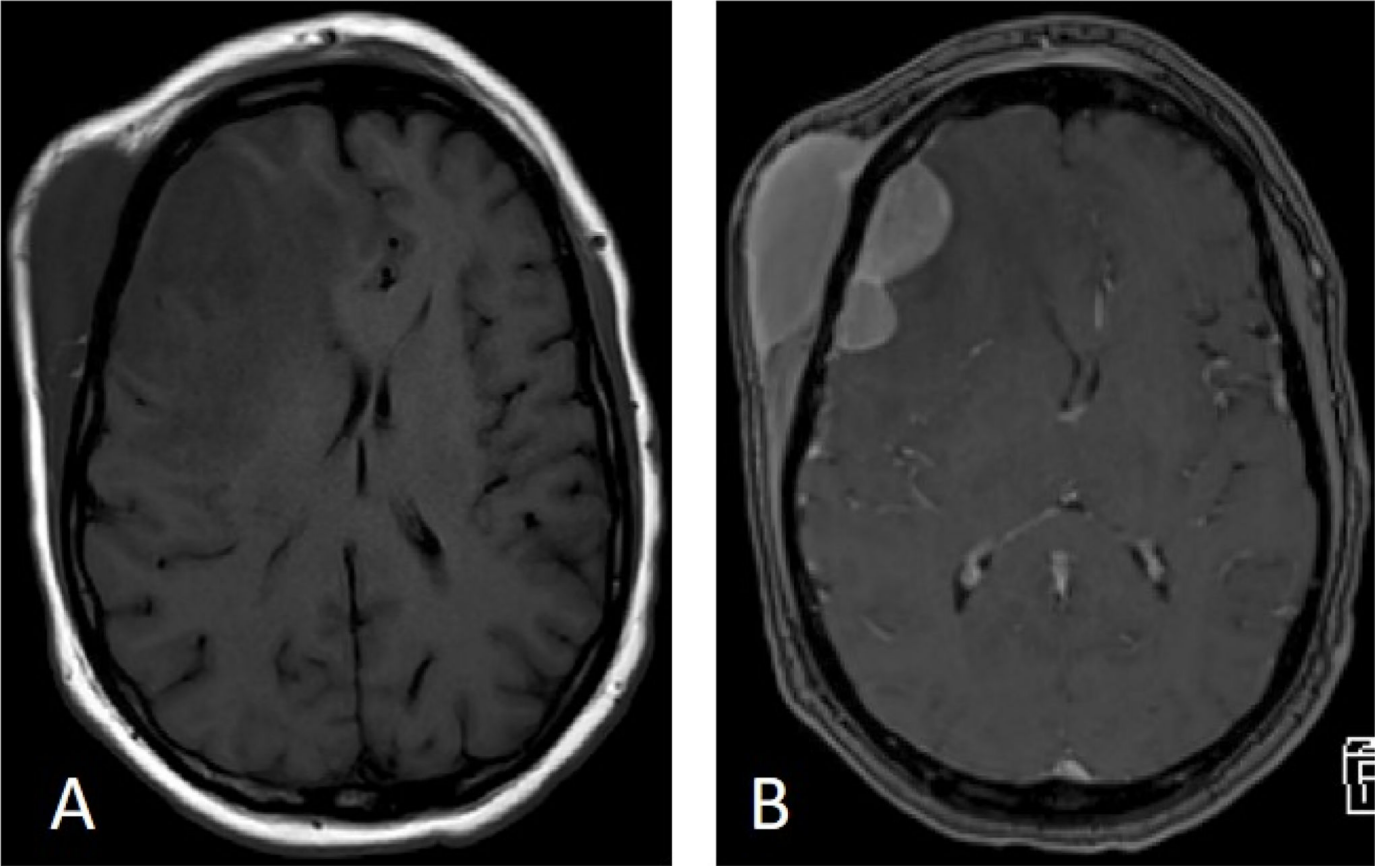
MRI showing scalp mass, vasogenic edema, and midline shift. A: Axial view T1 no contrast, B: Axial view T1 with contrast.
